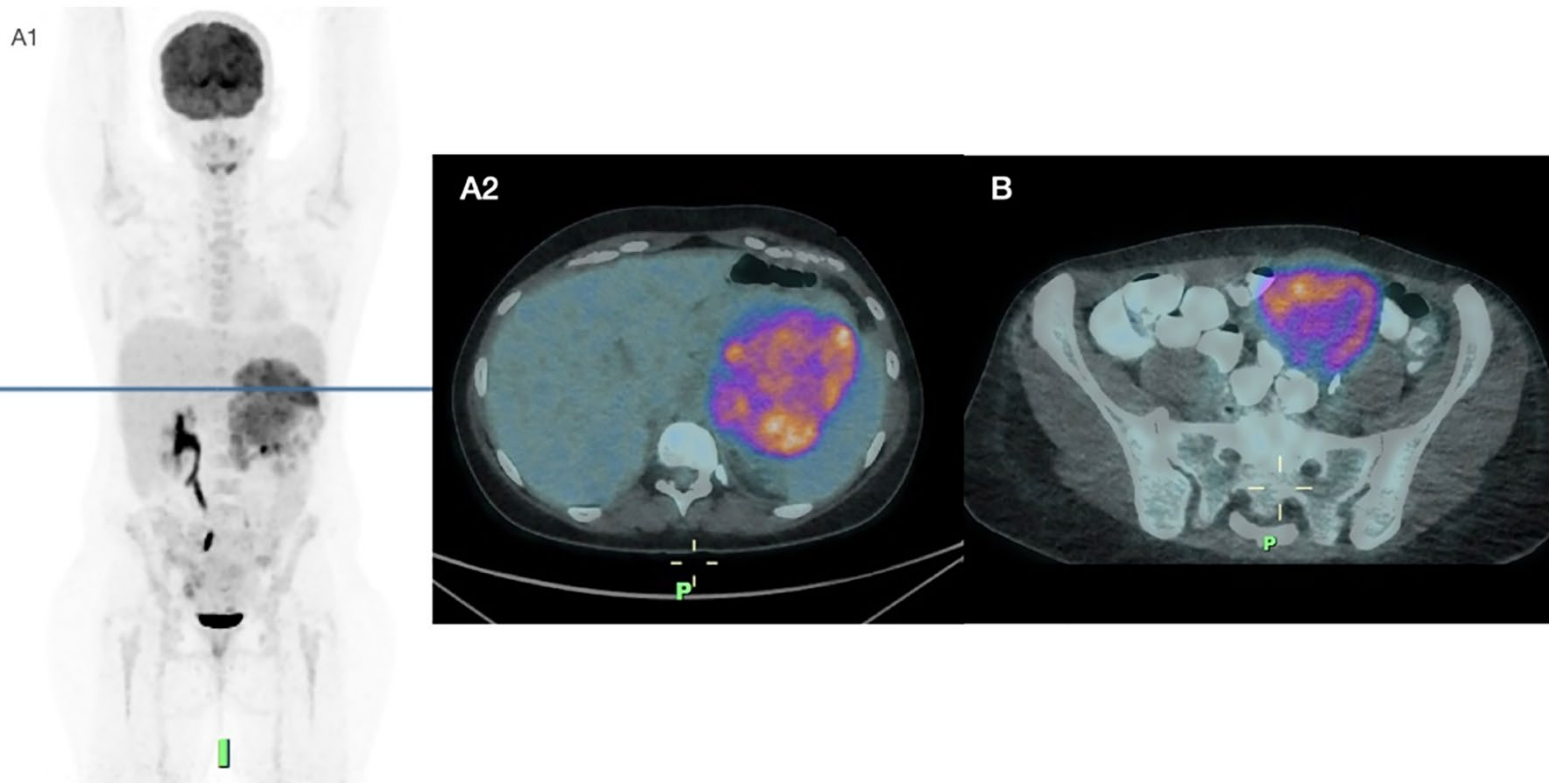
Preoperative staging with [^18^F]F FDG PET/CT(A1) in a 32-year-old patient with a finding of a voluminous left adrenal mass: (A2) axial PET/CT images show an intense and inhomogeneous uptake in the adrenal mass and in some lumbar aortic lymph nodes; after left nephrectosurrenectomy and left lymphadenectomy, a diagnosis of high-grade ACC was made. B At follow-up PET scan, during mitotane therapy, was found a peritoneal carcinosis
